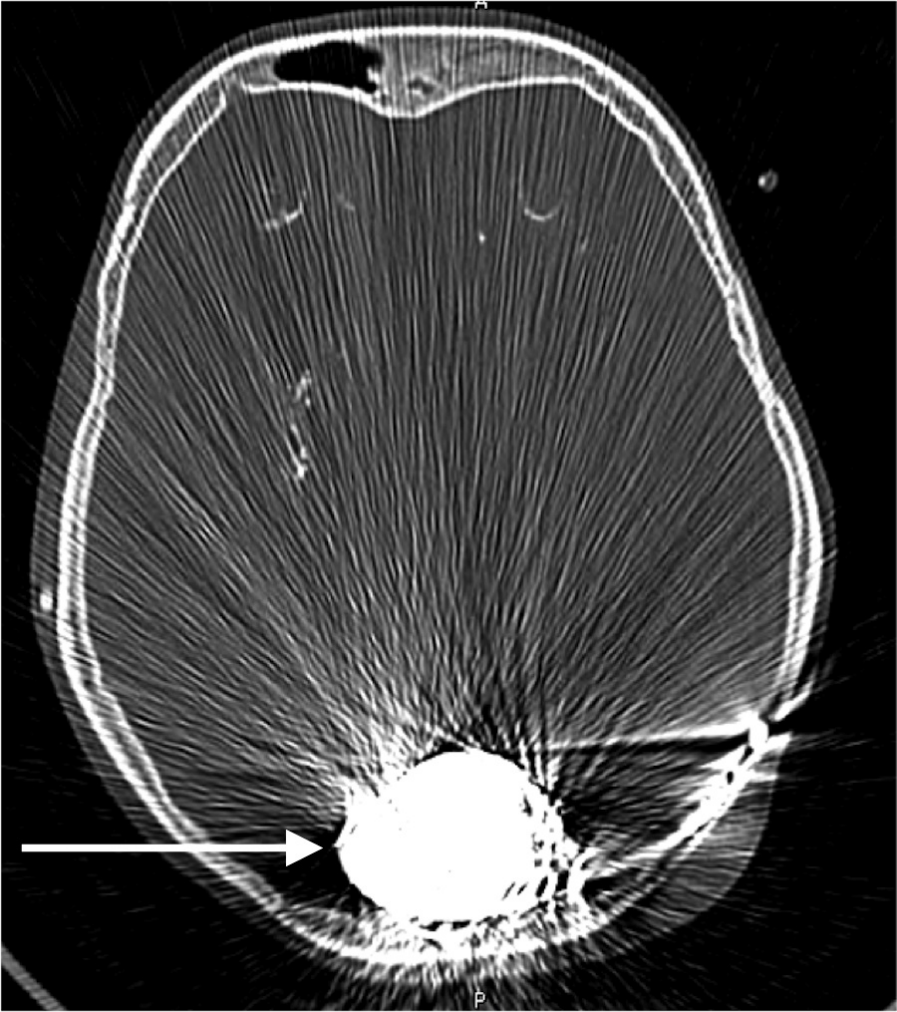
Post-operative computed tomography showing large coil mass (arrow)
